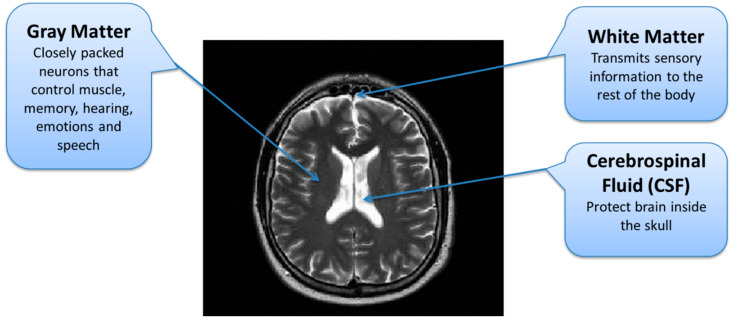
Brain MRI Tissues Types.
